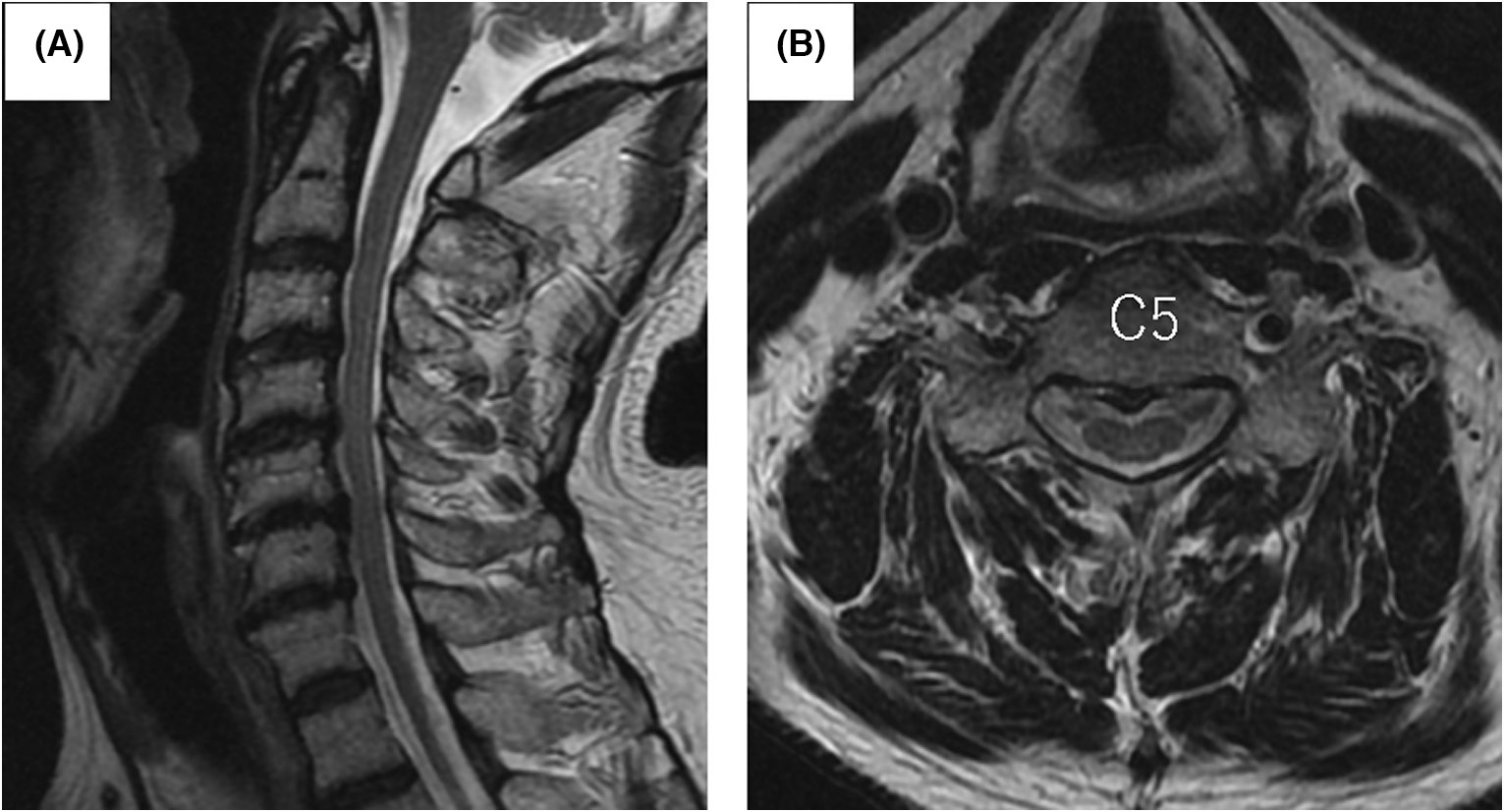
Magnetic resonance imaging of the cervical spine 6 months after treatment with subacute combined degeneration. Hyperintense signal changes in the spinal cord disappeared on both sagittal (A) and axial (B) MRI.
